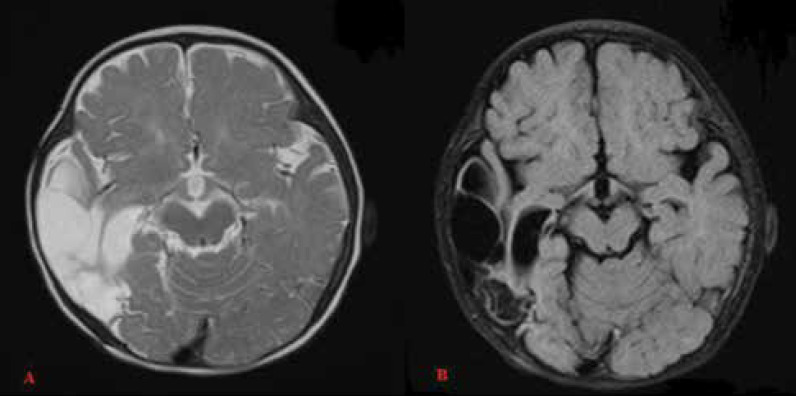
(A) Axial T2W MRI image, (B) Axial FLAIR MRI image, after tumor removal
